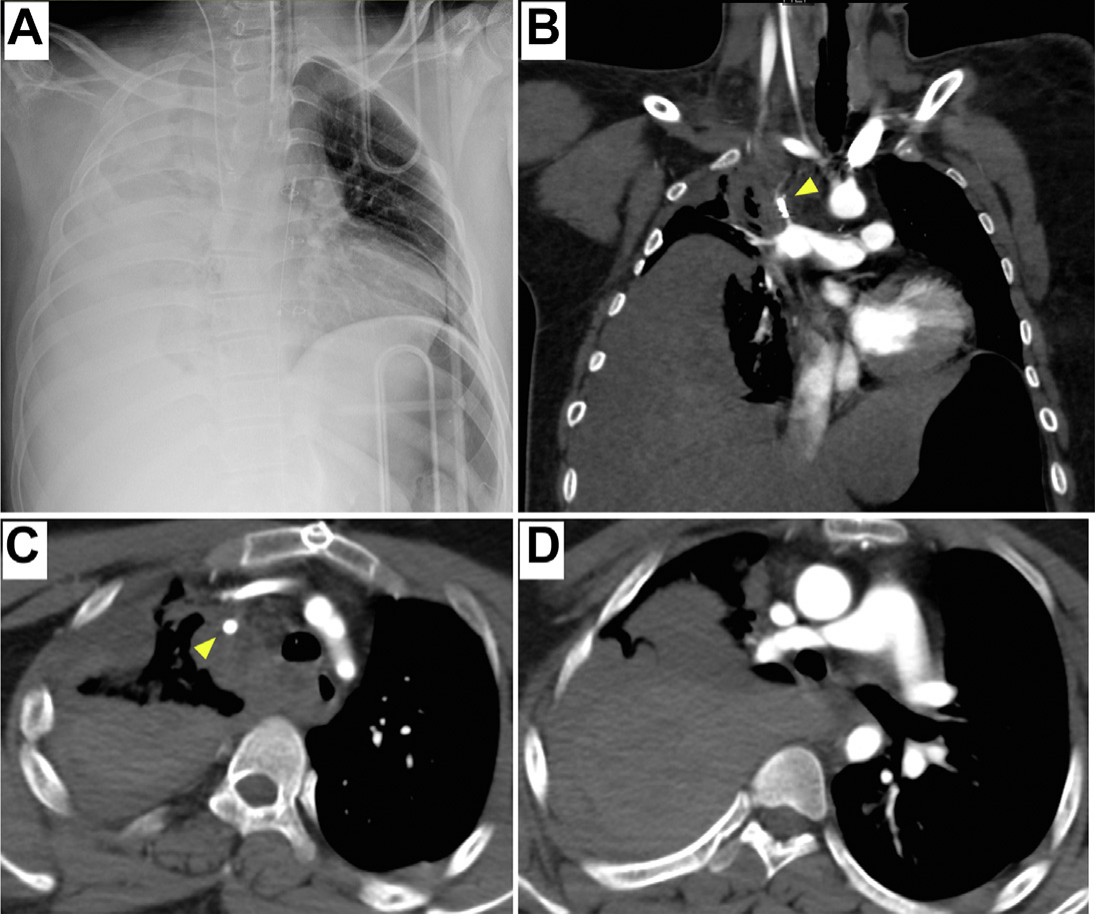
Radiologic images at admission. (A) Chest radiograph revealed no transparency in the right side of the chest, which implied hemothorax. (B-D) On chest computed tomography scans, subclavian artery side of Blalock-Taussig shunt (arrowhead) was disrupted, and fluid collection around it and right pleural effusion were observed.
